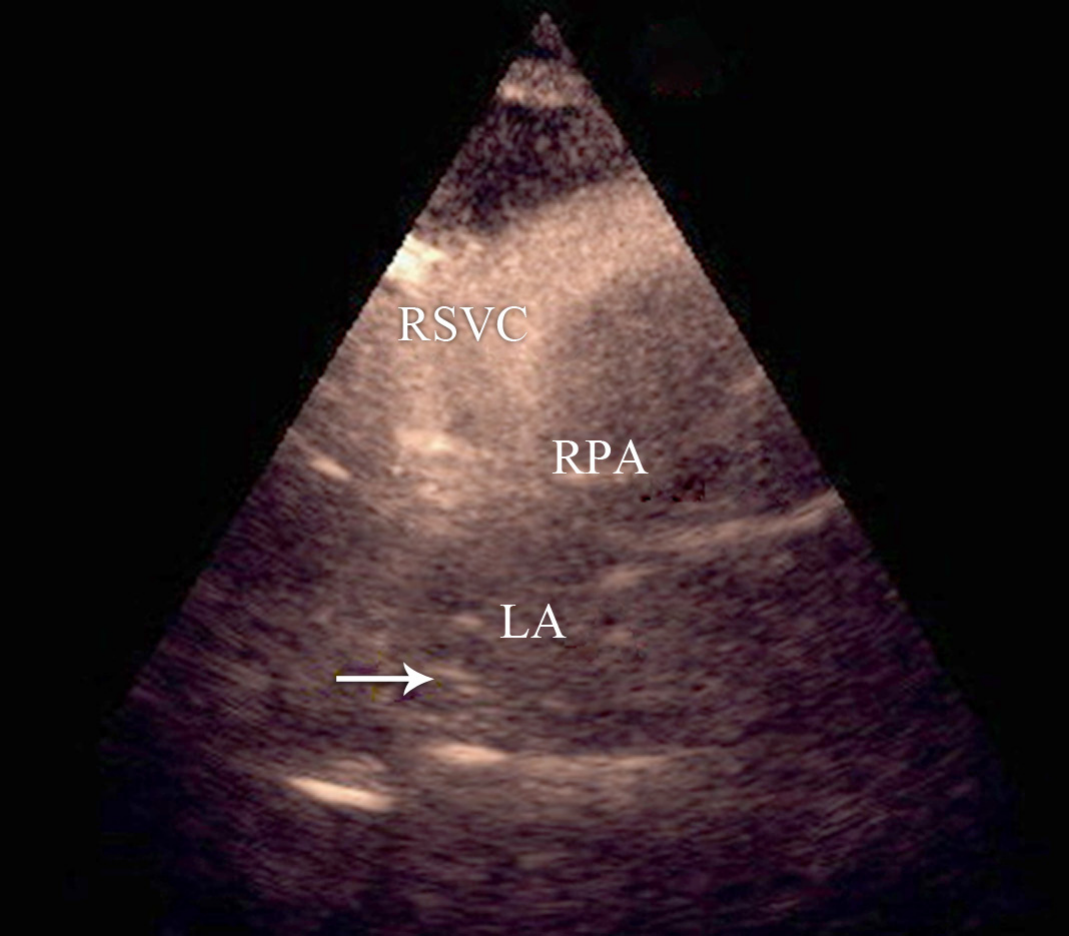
Transthoracic echocardiography in the suprasternal notch view, showing contrast within the dilated RSVC and the entrance of bubbles into the LA (arrow), ruling out an unroofed coronary sinus and a persistent left SVC.
